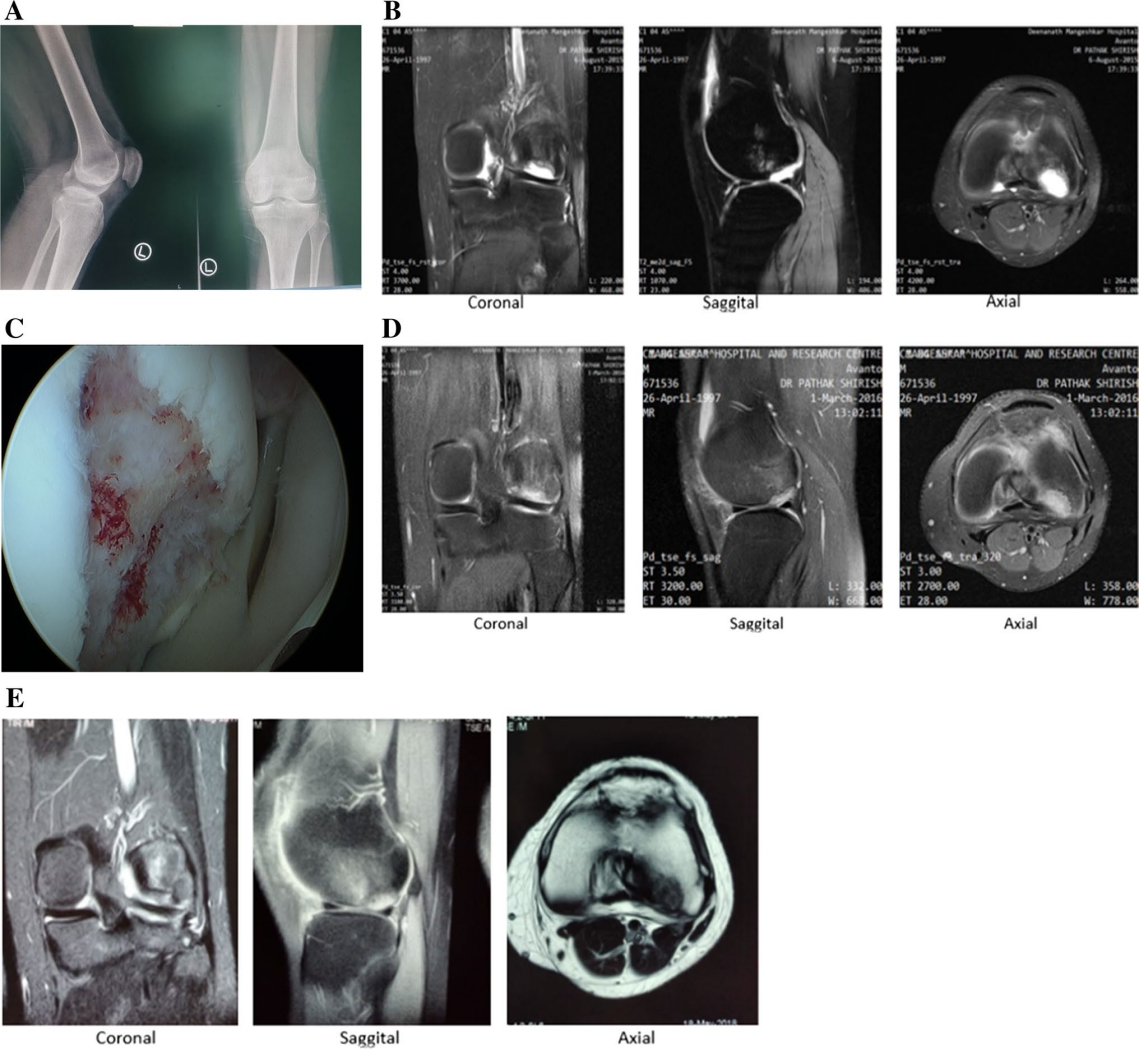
Pre-operative and post-operative clinical evaluations showing new cartilage formation in patient 2. A Preoperative X-ray of the left knee AP and lateral showing cartilage defect on left lateral femoral condyle. B Pre-operative MRI images of the same patient with PD-FS/T 2 coronal, sagittal and axial sections showing grade4 osteochondral defect over lateral femoral condyle. C Arthroscopic image showing ICRS grade 4 over lateral femoral condyle. D, E Post-operative MRI images at 6 months and 4 years of the same patient with PD-FS coronal, sagittal and axial sections showing good healing of cartilage defect post ACI, respectively
